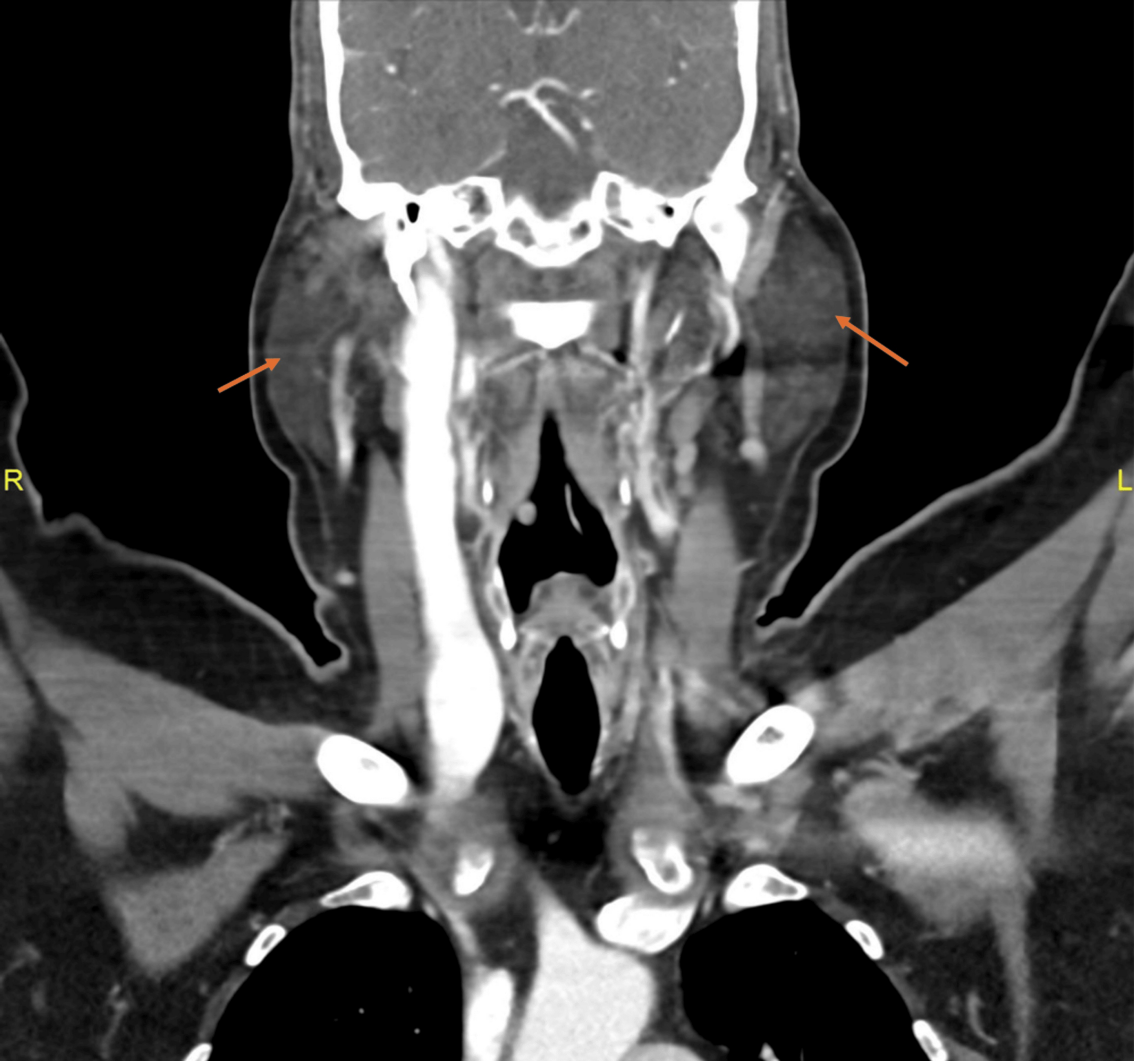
Sagittal CT scan of the head shows bilateral parotid gland enlargement.
